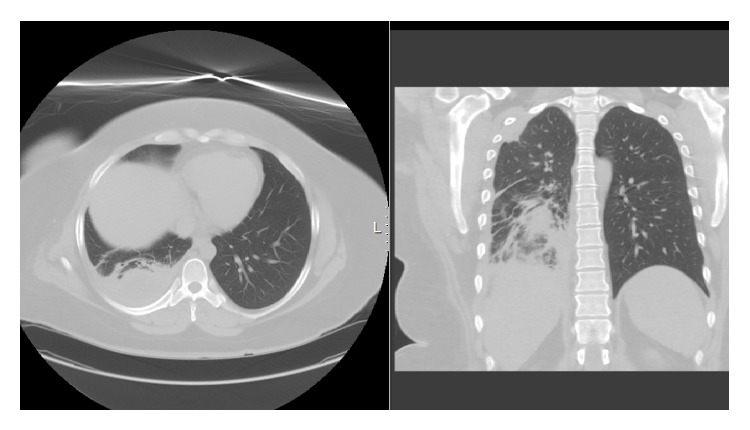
Chest CT on presentation.
